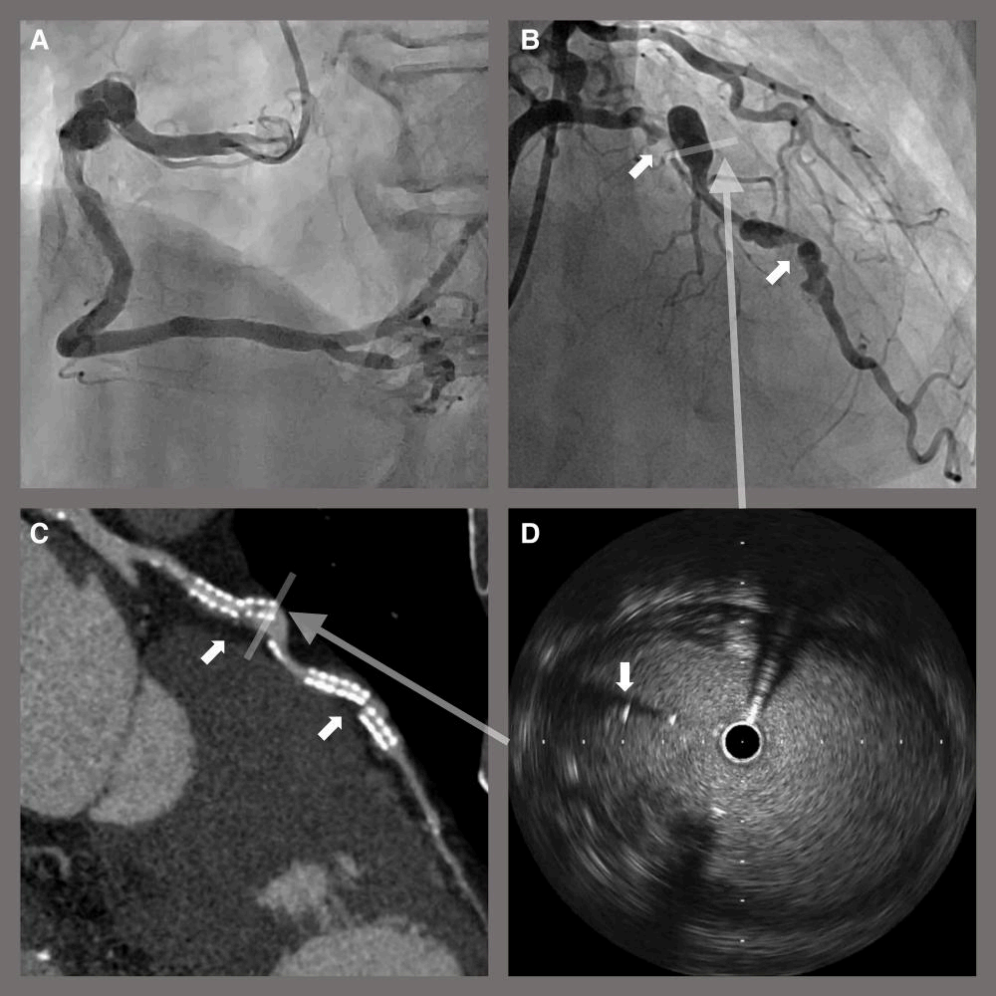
(*A*) Right coronary angiography revealed multiple aneurysm formation in sirolimus-eluting stent implanted site. (*B*) Left coronary angiography also showed multiple aneurysm formation in sirolimus-eluting stent implanted site. Moreover, restenosis and stent fractures (arrows) were observed. The grey line perpendicular to the vessel at the end of the long grey arrow indicates the area where the intravascular ultrasound image in *D* was obtained. (*C*) Computed coronary angiography of left anterior descending artery showed stent fractures (arrows). The grey line perpendicular to the vessel at the end of the long grey arrow indicates the area where the intravascular ultrasound image in *D* was obtained. (*D*) Intravascular ultrasound images showed true aneurysm with a maximum size of 7 × 10 mm at the distal end of the proximal sirolimus-eluting stent in the left anterior descending artery. An arrow indicates the stent.
